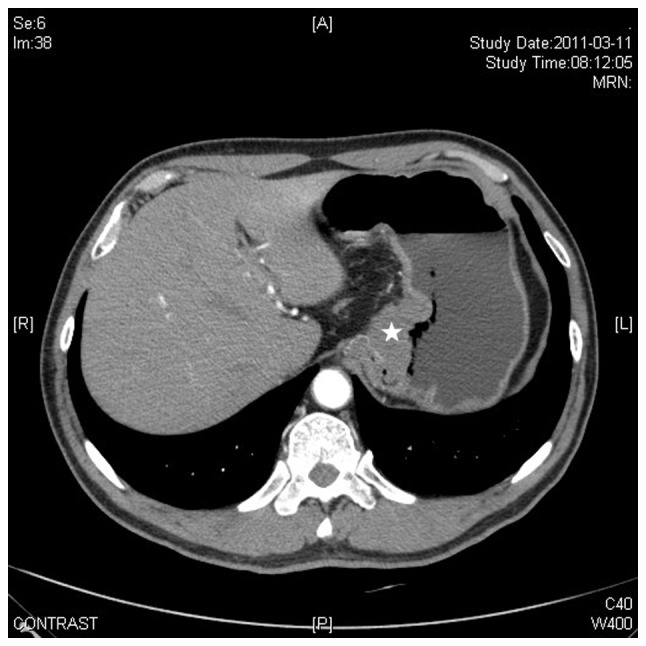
Enhanced computed tomography scan showing the abnormally thickened stomach wall of the cardia (indicated by the star symbol). The lymph nodes around the lesser curvature were enlarged.
